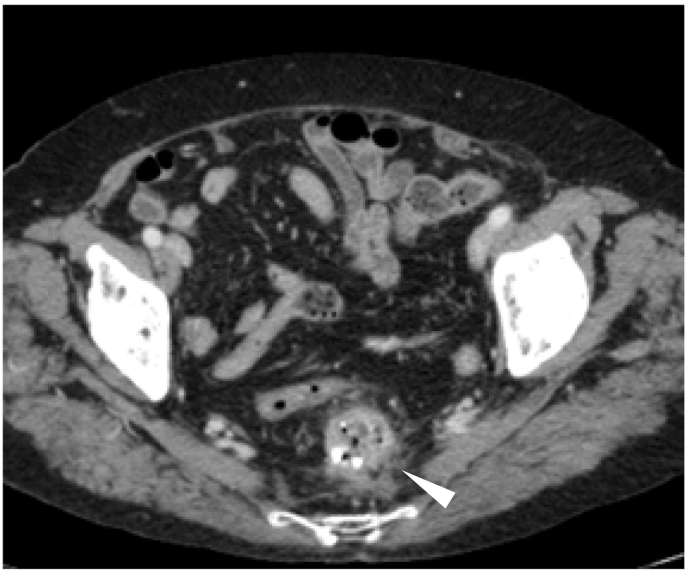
Computed tomography showed a diverticulum-like structure with panniculitis in the fat tissue behind the middle rectum, and a high-density structure was identified inside the diverticulum-like structure (white arrowhead).
